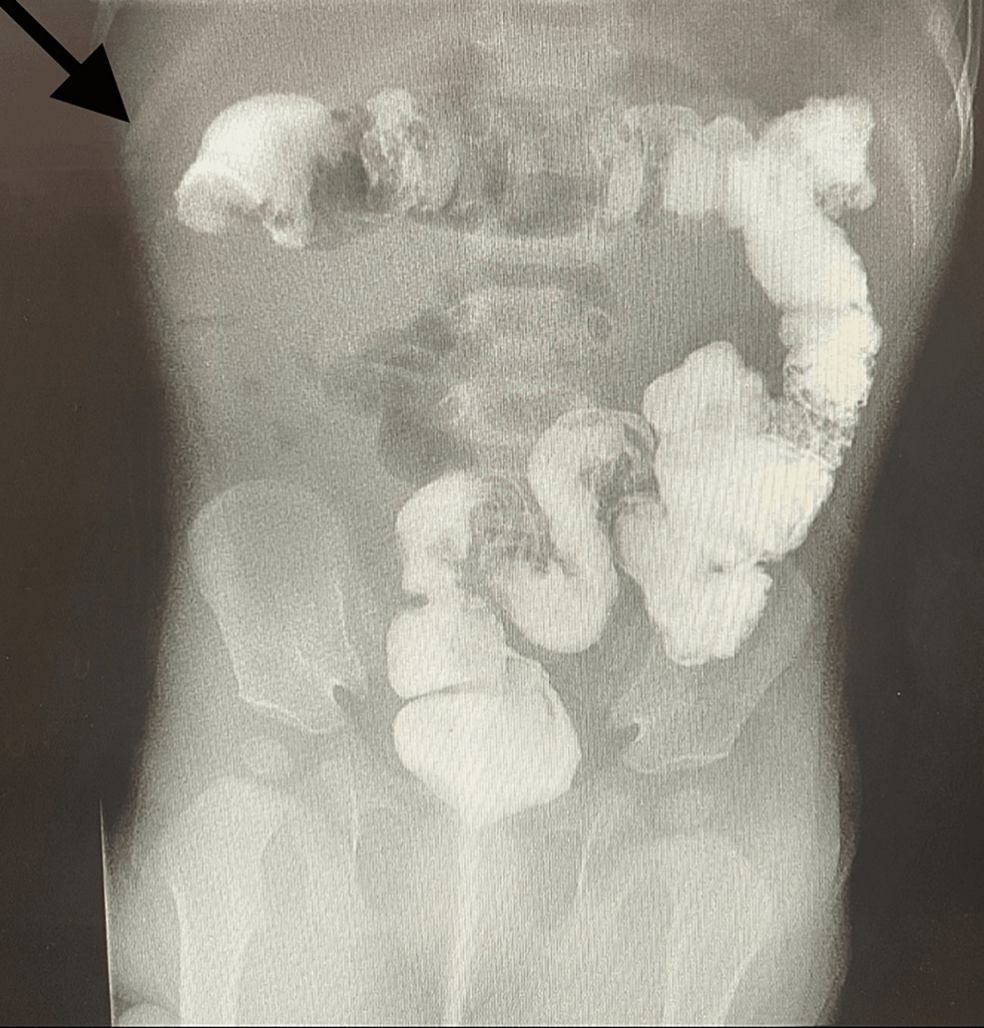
Day 1 X-ray gastrografin enema image showing the obstruction at the hepatic flexure
